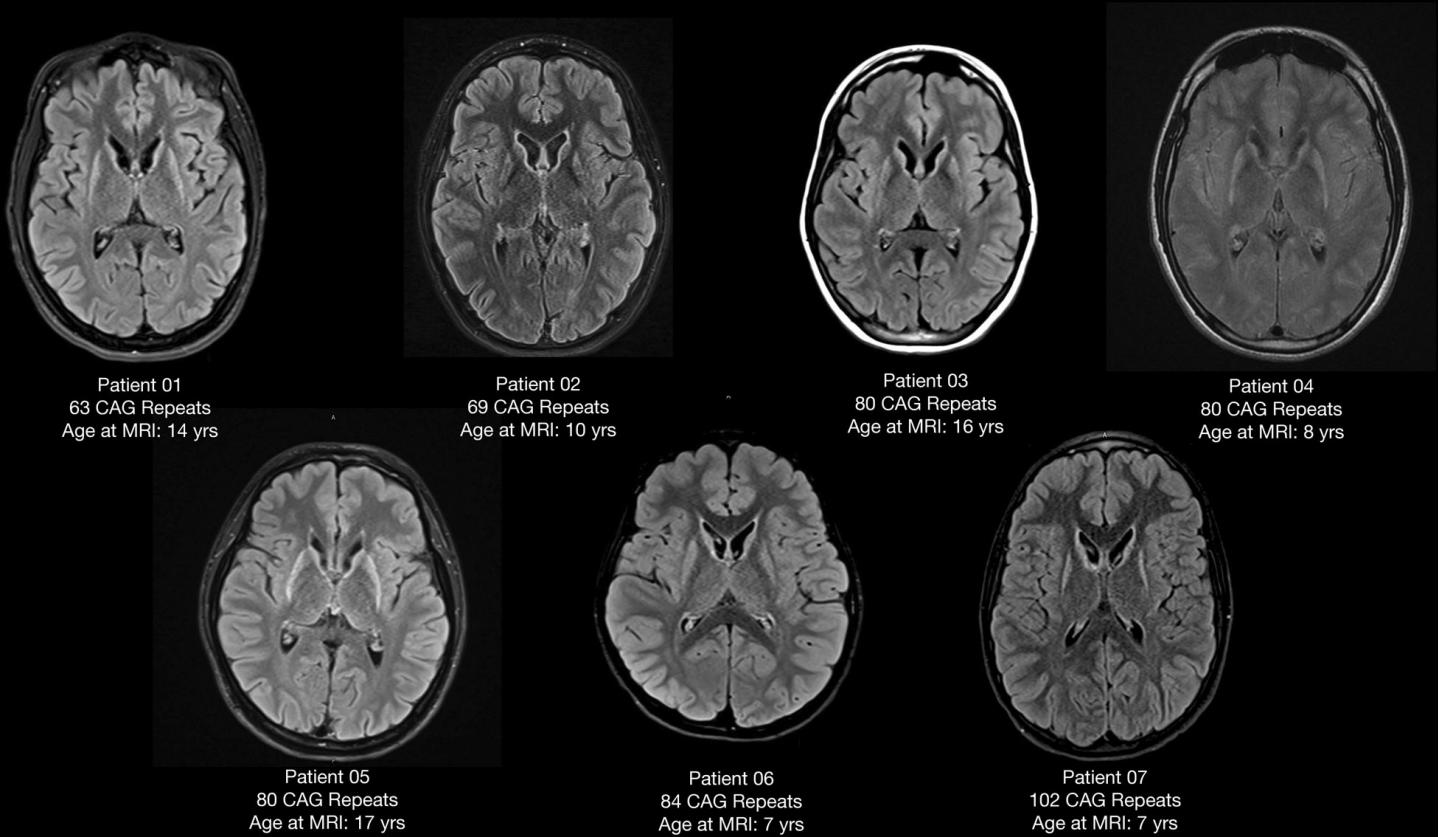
Brain MR imaging with representative axial T2‐FLAIR images demonstrating the universal feature of atrophy and signal prolongation in the caudate and putamen bilaterally.
